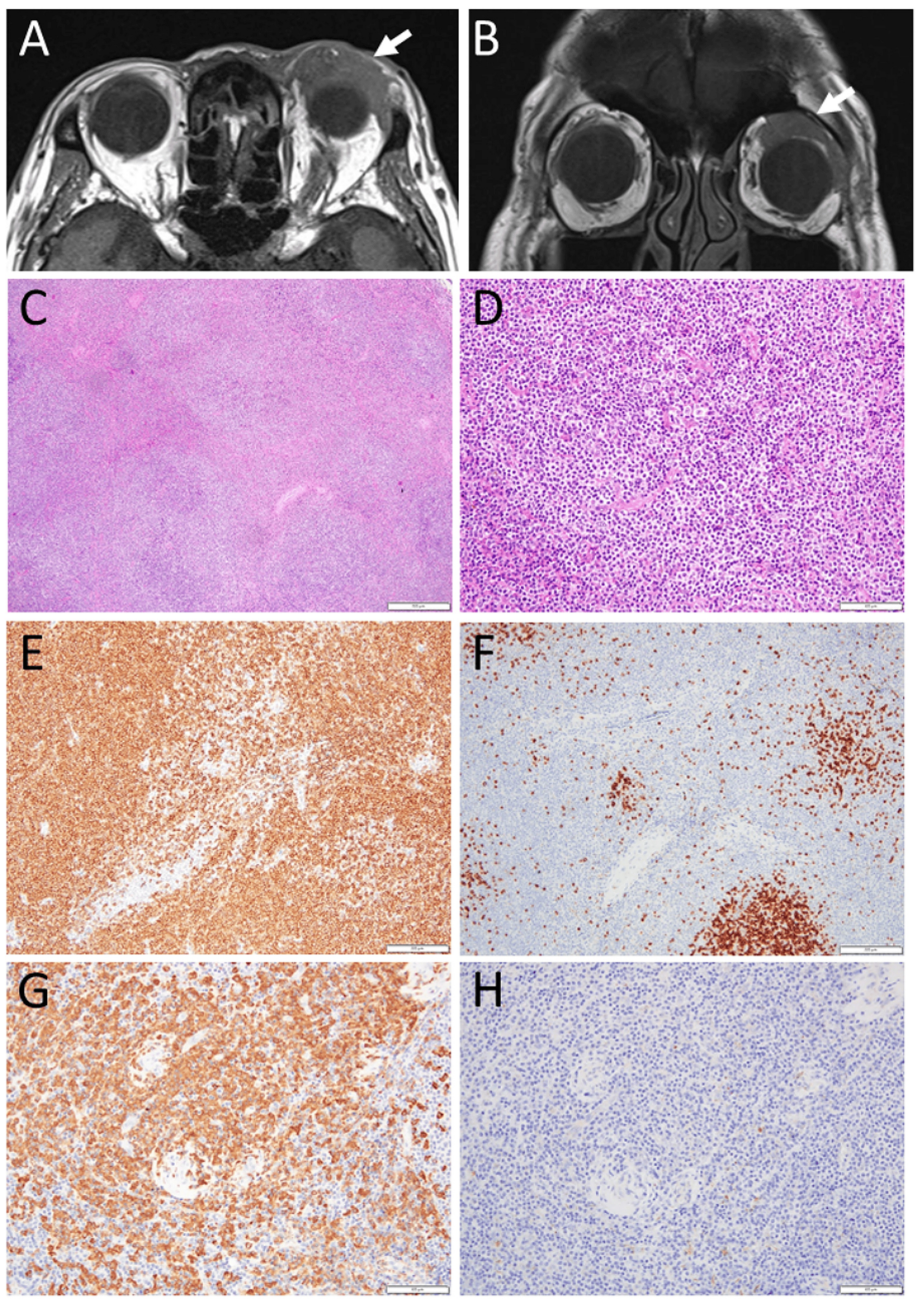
Left orbital mass lesion. Magnetic resonance imaging at the age of 77 years, showing a left superior anterior orbital mass (arrows) in T1-weighted axial (A) and coronal (B) images. Resection of left orbital mass, leading to the diagnosis of extranodal marginal zone B-cell lymphoma of mucosa-associated lymphoid tissue (MALT lymphoma) at the age of 78 years. Ambiguous nodular structure (C) with massive infiltration with medium-sized lymphoid cells (D). Most of lymphoid cells are positive for CD20 (E), admixed with small foci of CD3-positive cells (F). By in situ hybridization, lymphoid cells are all positive for immunoglobulin light chain κ (Igκ, G), and all negative for immunoglobulin light chain λ (Igλ, H), indicating immunoglobulin light chain restriction to κ (κ monotype). White scale bar = 500 µm in C, 100 µm in D, G, H, 200 µm in E, F.
